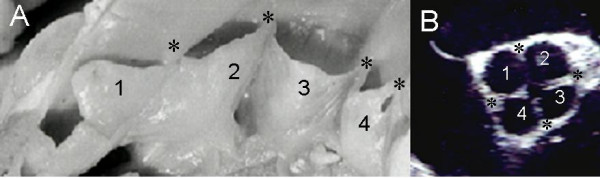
Quadricuspid aorta. Photograph (A) and transesophageal image at 50° (B) of aortic valves with four leaflets (1,2,3, and 4) and four commissures (asterisks).
